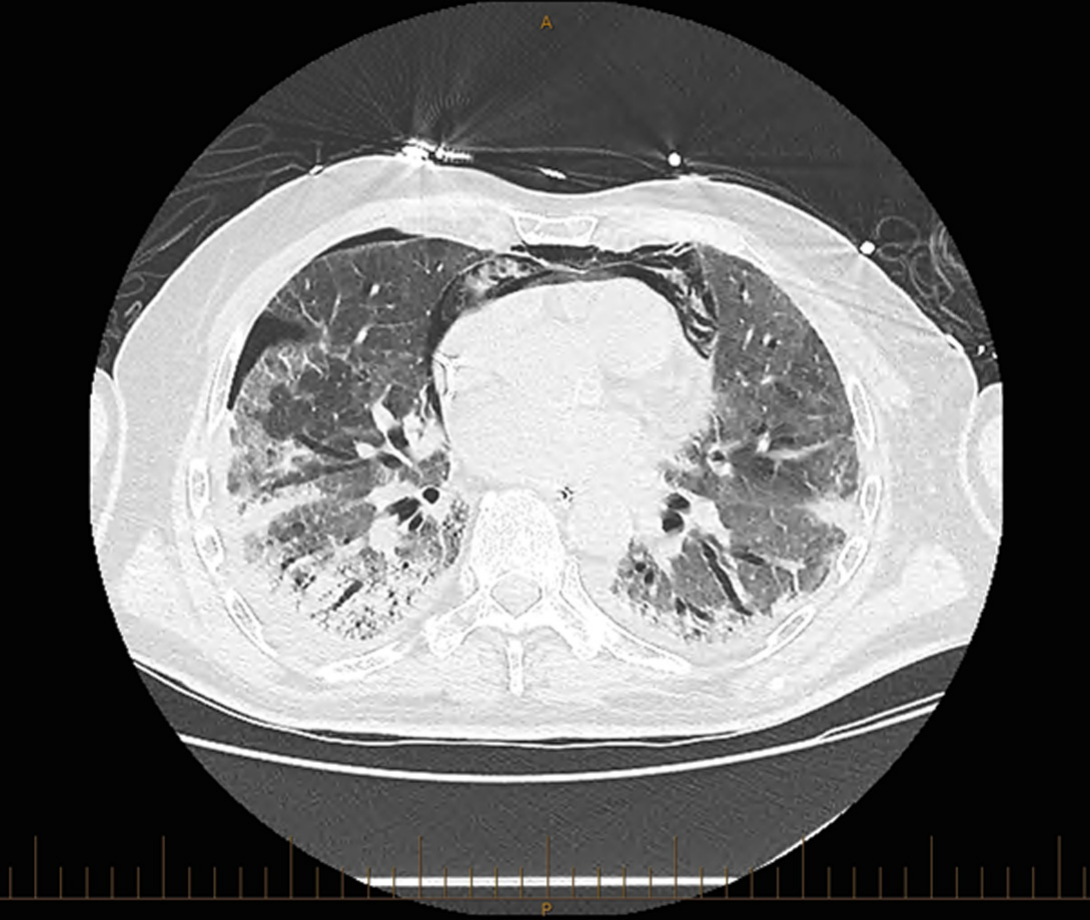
CT scan on day 3 showing a pneumomediastinum and a small right pneumothorax.
